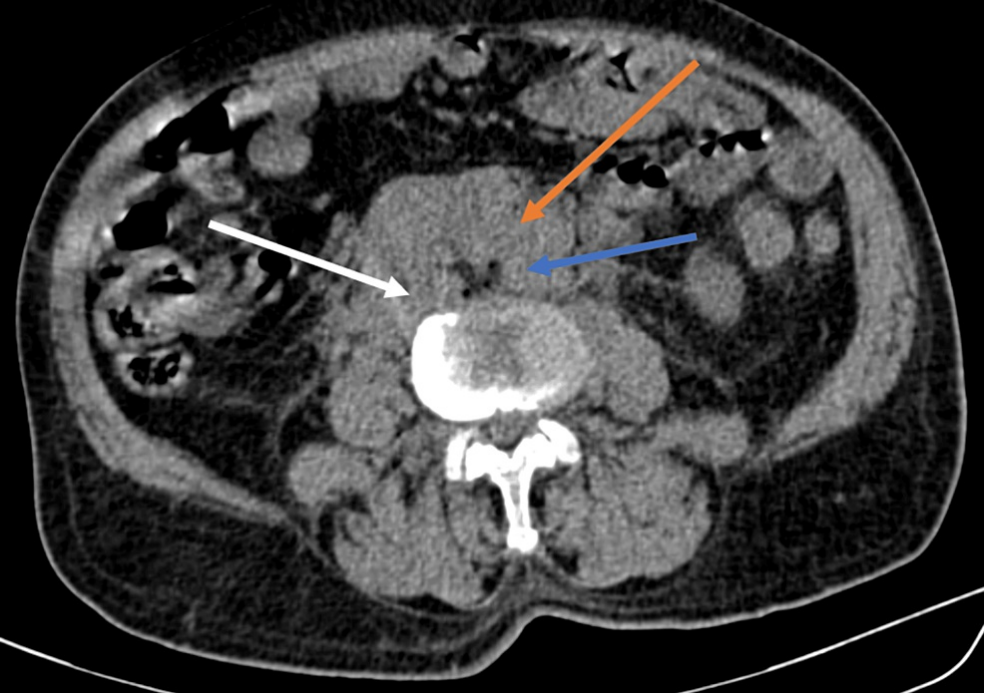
Transverse section of computed tomography of kidney, ureter, bladder showing a large multilobulated soft tissue density mass (Orange arrow) noted in retroperitoneum completely encasing aorta (Blue arrow) suggestive of a conglomerated lymph nodal mass. The mass is also causing variable external mass effect/encasement of mid-portion of both ureters leading to bilateral proximal moderate hydroureteronephrosis. White arrow indicates inferior vena cava.
